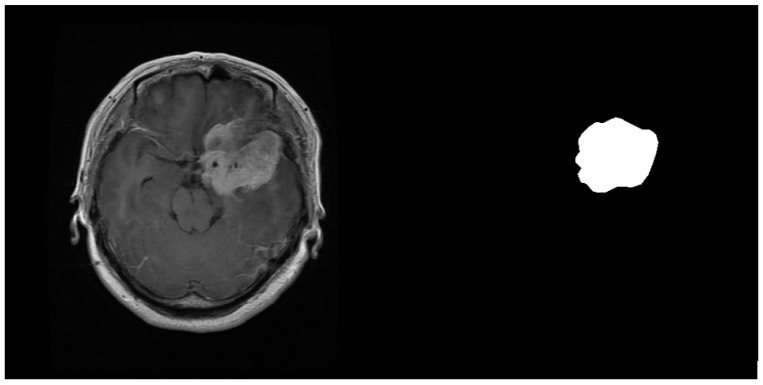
Example MRI image and corresponding tumor mask from the dataset.
